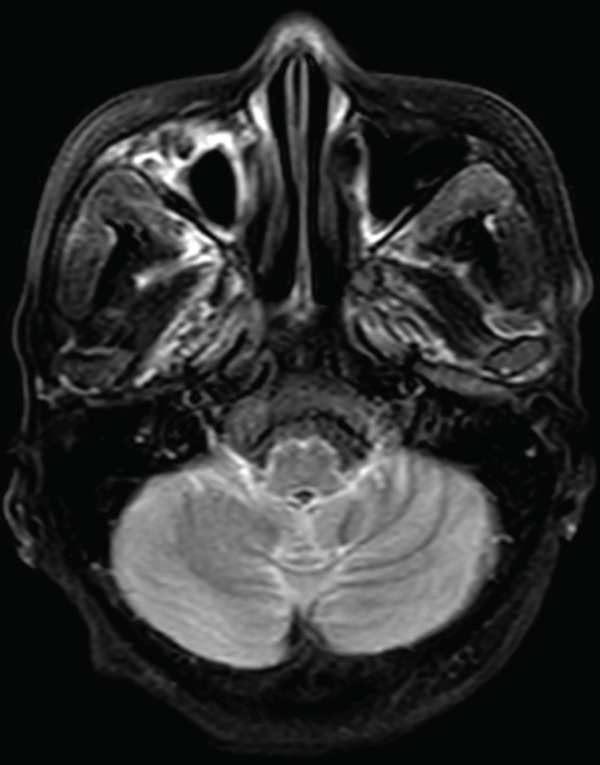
Linear enhancement in the cerebellar hemisphere on MRI.
